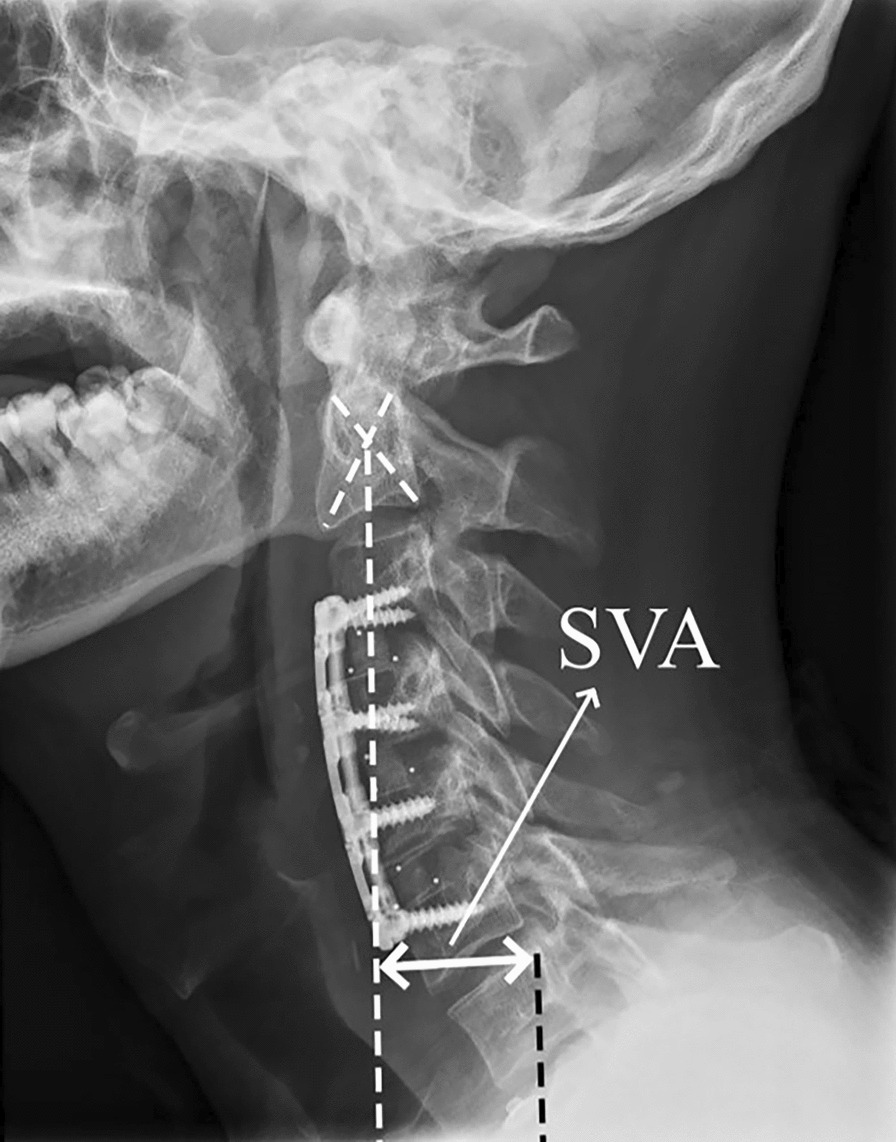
Postoperative three-segment ACDF X-rays also show SVA measurements (the distance between a plumb line dropped from C2 and the posterior–superior corner of C7)
